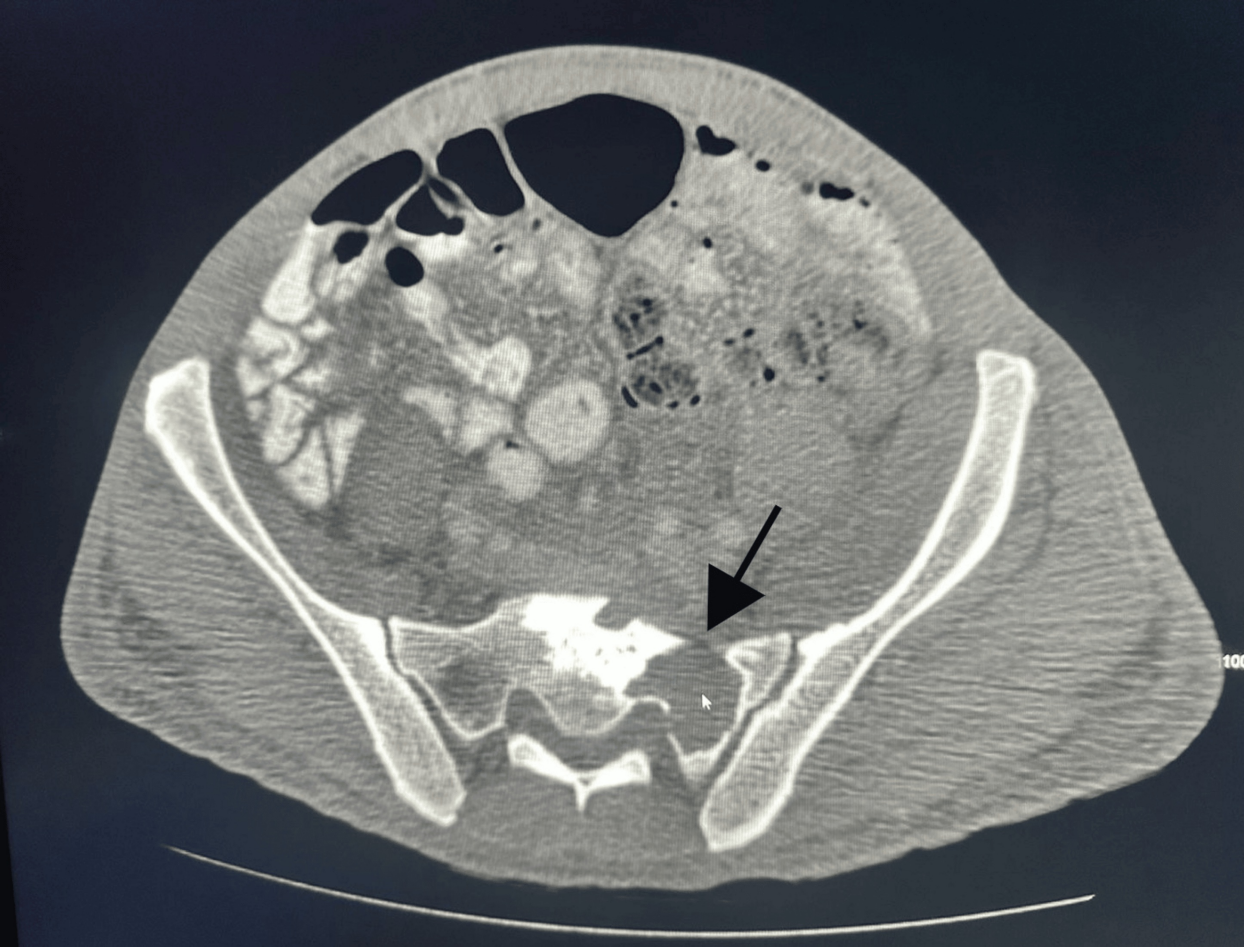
CT of the abdomen and pelvis showing a large lytic lesion in the left sacral bone (black arrow). CT: computed tomography.
